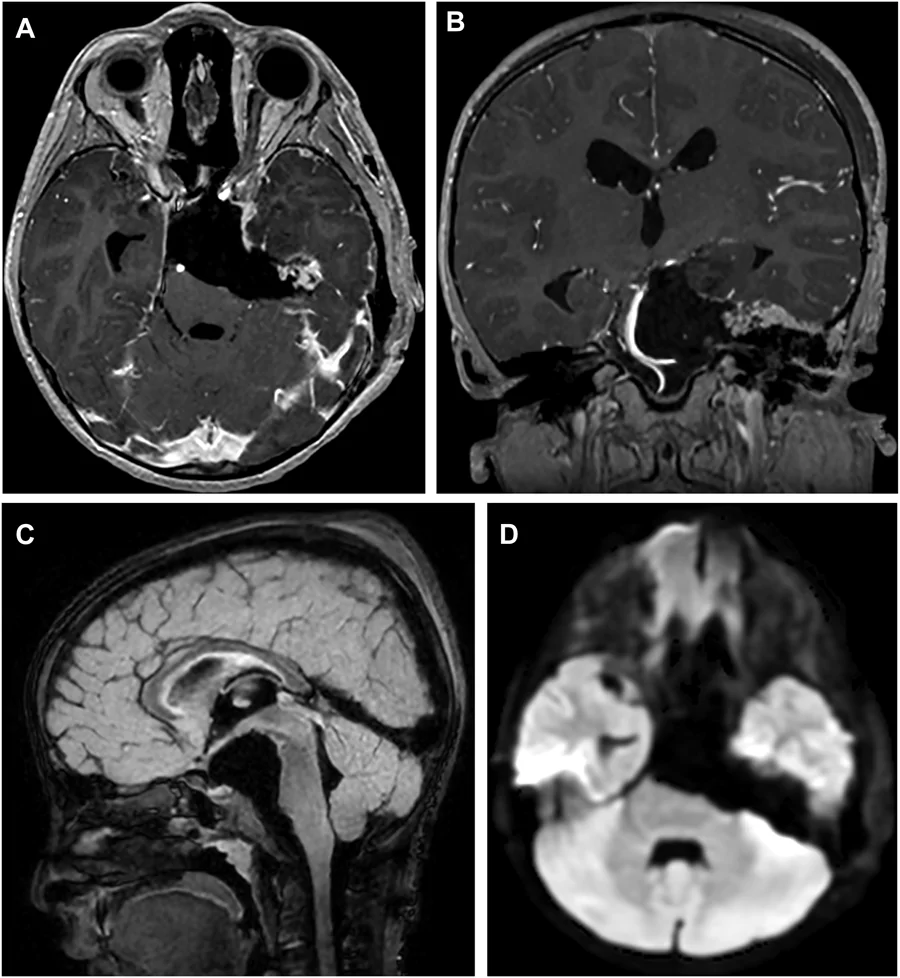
Postoperative MRI demonstrates complete resection of the dermoid cyst. Axial (A) and coronal (B) T1-weighted images and sagittal fluid-attenuated inversion recovery (C) show resolution of brainstem compression without residual lesion. Diffusion-weighted imaging (D) reveals no restricted diffusion, consistent with the complete removal of dermoid content. This figure is original to this submission, so no credit or license is needed.
